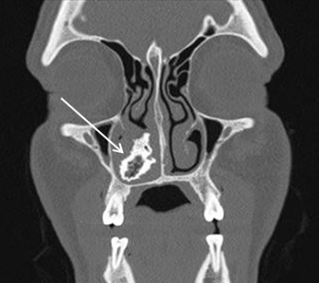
Coronal computed tomography demonstrating calcified mass indicated by white arrow in the inferior meatus.
